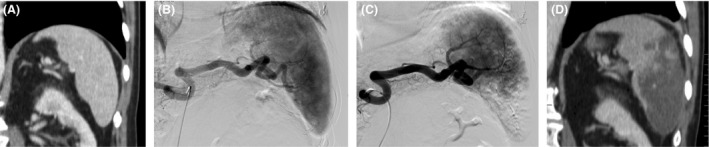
(A) Computed tomography showing enlarged spleen in a patient with colorectal cancer after 7 cycles of Folfox. (B) Pre‐embolization arteriogram showing usual splenic arterial anatomy. (C) Immediate post partial splenic embolization (PSE) arteriogram showing embolization of approximately 50% of the splenic parenchyma (D) Computed tomography 2 weeks after PSE showing partial spleen necrosis, which accurately correlated with the post PSE arteriogram.
